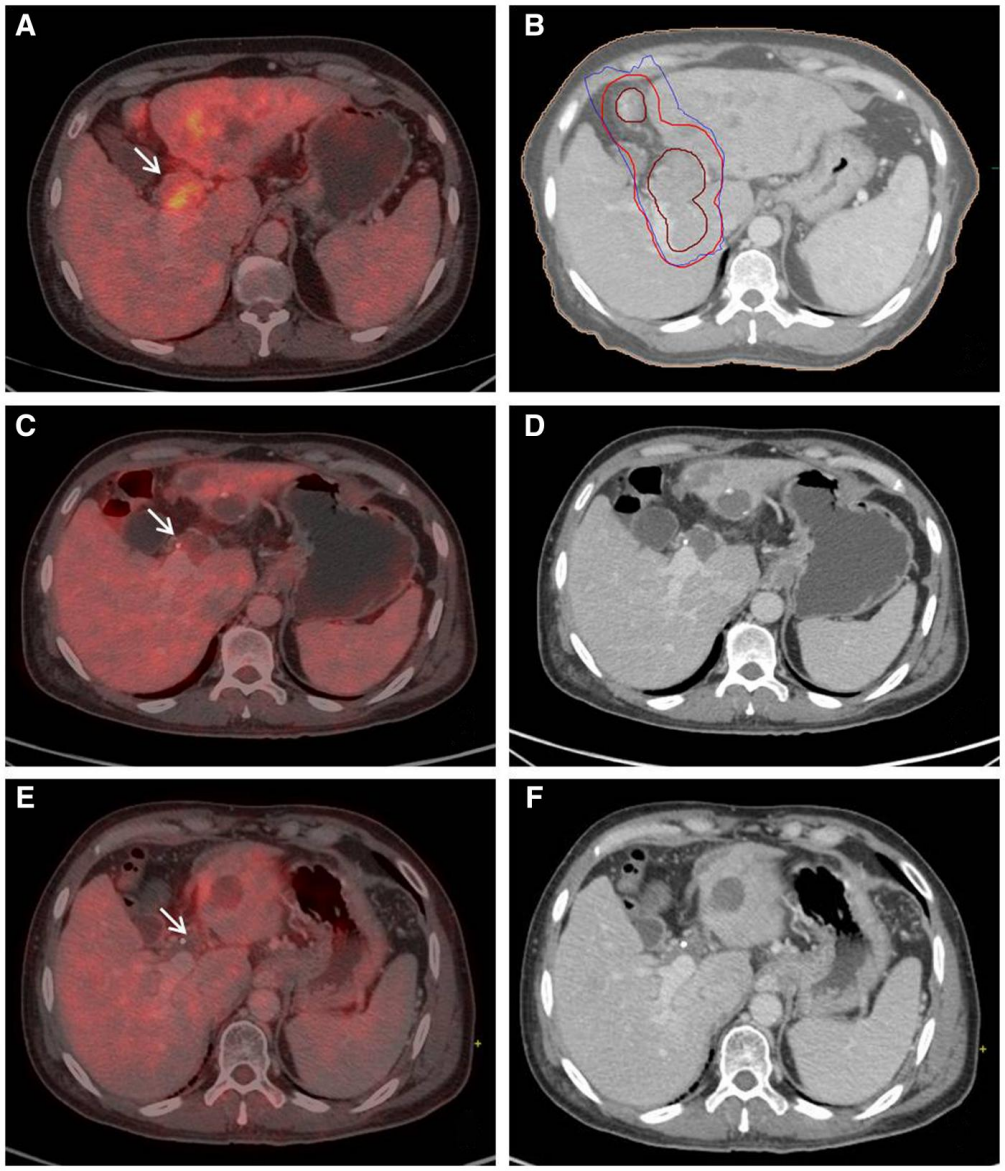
(A) Axial image of fused 18F-FDG PET-CECT shows FDG avid portal vein tumour thrombus (PVTT) (arrow) with lesion in left lobe; (B) Axial image of radiotherapy planning CECT on venous phase shows gross tumour volume delineation of PVTT along with planning target volume covered with 100% isoline; (C) and (D) Axial images of fused 18F-FDG PET-CECT and venous phase of CECT at 3 months after radiotherapy, systemic treatment, and DEB-TACE show FDG resolution and shrinkage of PVTT (arrow) with post DEB-TACE changes in left lobe lesion; (E) and (F) Axial images of fused 18F-FDG PET-CECT and venous phase of CECT at 1 year after radiotherapy with systemic targeted therapy and DEB-TACE shows complete response. Abbreviations: 18F FDG PET-CECT = 18Fluorine fluorodeoxyglucose PET–contrast-enhanced CT; CECT = contrast-enhanced CT; DEB-TACE = drug eluting beads transarterial chemoembolization.
